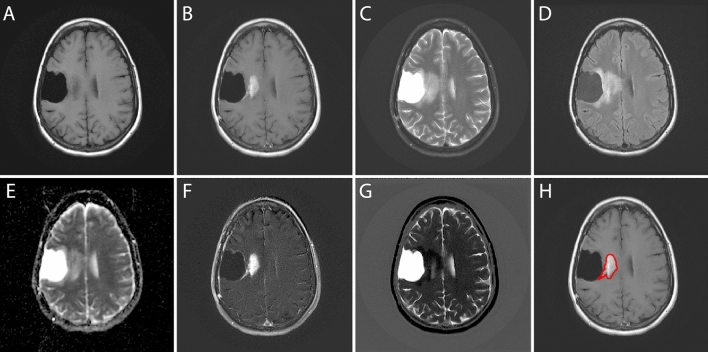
A GBM patient (PsP) with various MRI scans such as (A) pre-contrast T1-weighted, (B) post-contrast T1-weighted, (C) T2-weighted, (D) Fluid-Attenuated Inversion Recovery (FLAIR), and (E) ADC images. In this study, we included two engineered modalities (F) T1post–T1pre and (G) T2–FLAIR. And (H) the region of interest (ROI) is shown on a post-contrast T1 image.
